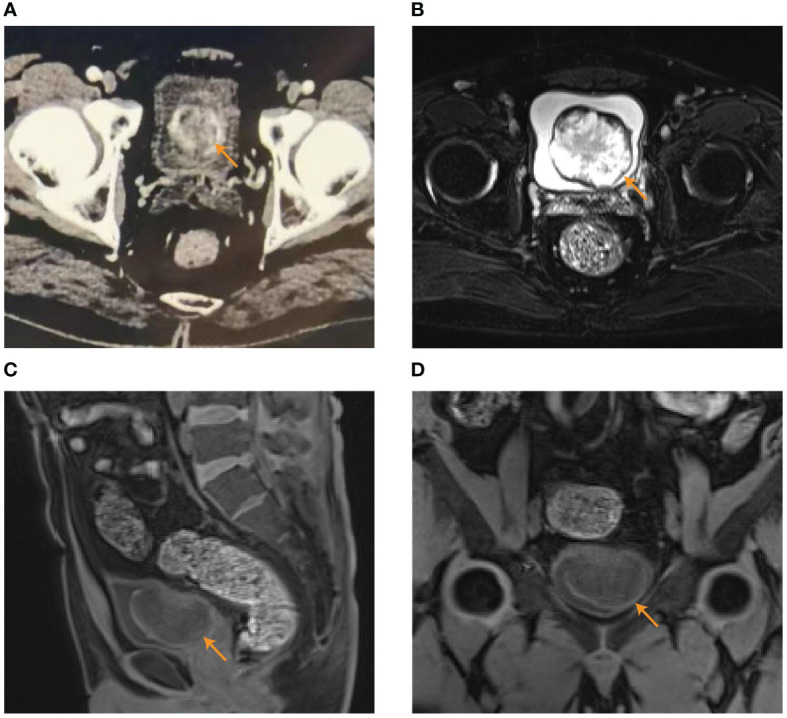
The results of preoperative examination. (A) CTU indicated a space occupying lesion (6.0 cm×5.0 cm) in the left posterior wall of the bladder with heterogeneous enhancement in the excretory phase. (B–D) MRI indicated bladder tumor (6.0 cm×5.1cm×3.5cm) with mixed high SI on T2WI in transverse plane (B) and slightly equal SI on T1WI in sagittal position (C) and coronal position (D).
